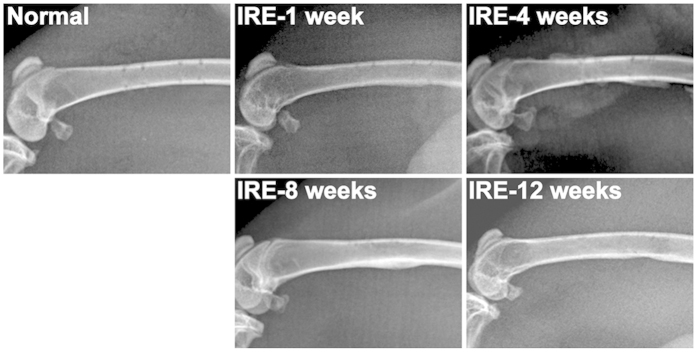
X-ray observation of the IRE-ablated bone in the corresponding period. Normal: The electrode holes were visible. IRE-1 week: A fibrous callus packed the holes, and a thin layer of calcified callus formed in the posterior of the femur. IRE-4 weeks: The callus in the outer layer of the cortex thickened, and a new callus filled the electrode holes. IRE-8 weeks: The callus thinned. IRE-12 weeks: The callus almost disappeared.
